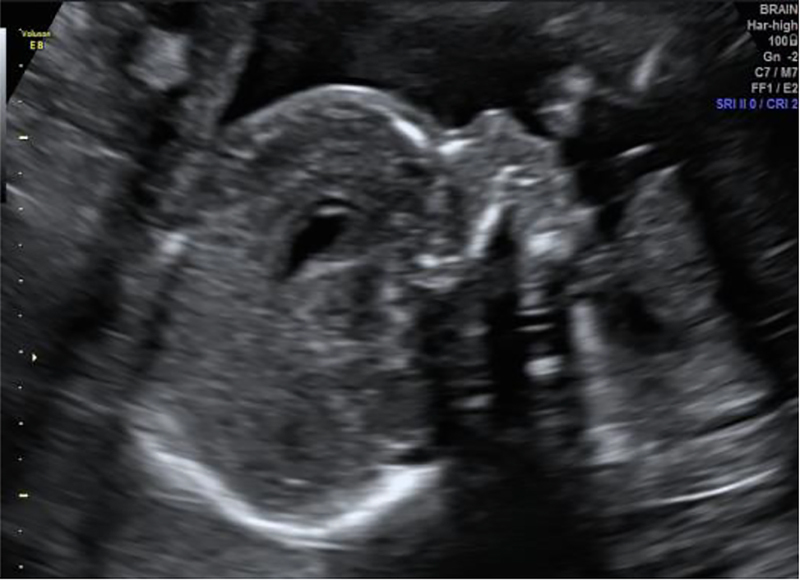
Ultrasound at 21 weeks revealing a significant improvement of amniotic fluid index (oligohydramnios).
